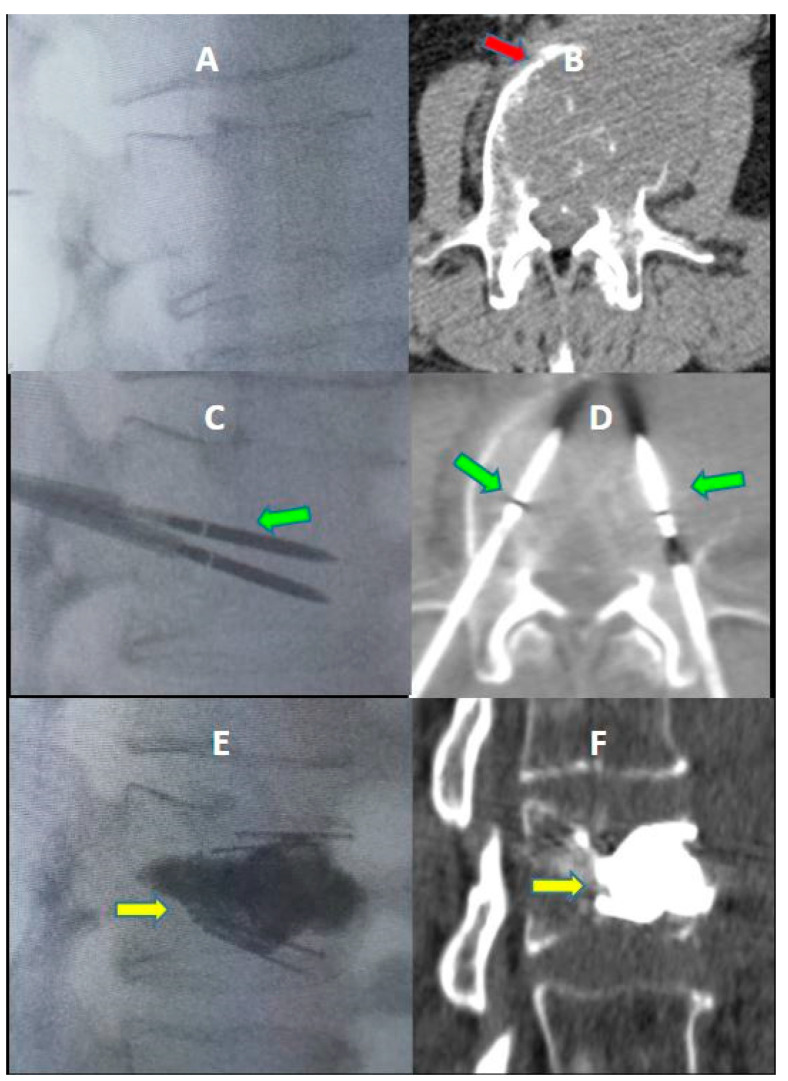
A 58-year-old male with a history of colon cancer with lumbar pain. (A,B) lateral fluoroscopic image—Axial CT image showed a pathological compression fracture of the superior endplate of the vertebral body of L3 with extension to the periosseous soft tissue (red arrow in (B)); (C,D) lateral fluoroscopic image ©—axial CT image (D); microwave antennae inserted coaxially through cannulae for ablation (green arrows); (E,F) lateral fluoroscopic image (E)—axial CT image (F); post-procedure control showed a correct expansion of the vertebra with a homogeneous distribution of cement into the vertebral body (yellow arrows). No procedural complications were observed.
